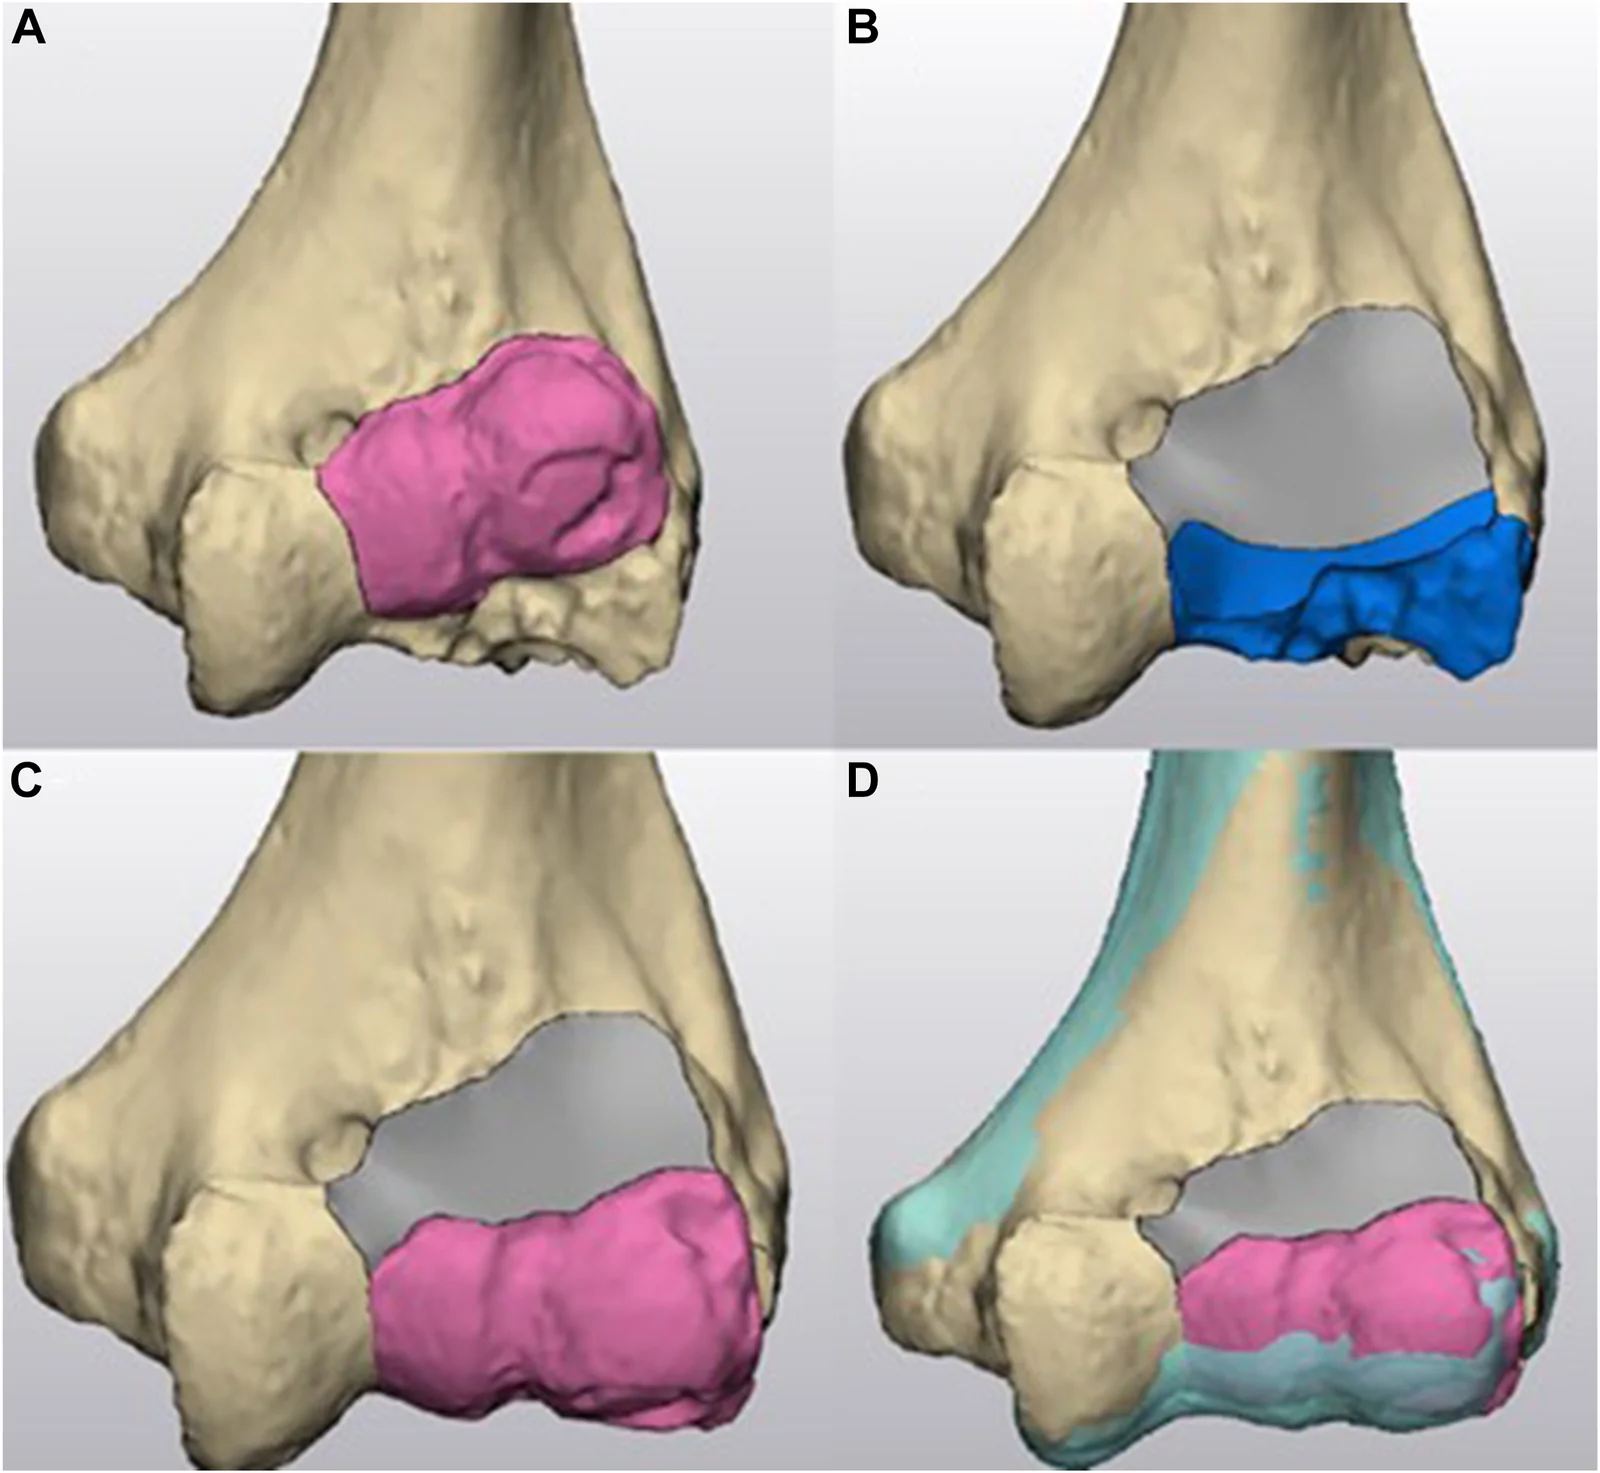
Case 1 - Pre-operative 3D CT reconstruction of the elbow joint. (A) Malunited capitellum highlighted in pink. (B) Highlighted in blue, the additional bone to be resected before reduction of the capitellar fragment. (C) Capitellar fragment after reduction. (D) Comparison with contralateral distal humerus (light blue) after reduction. *3D*, three-dimensional; *CT*, computed tomography.
